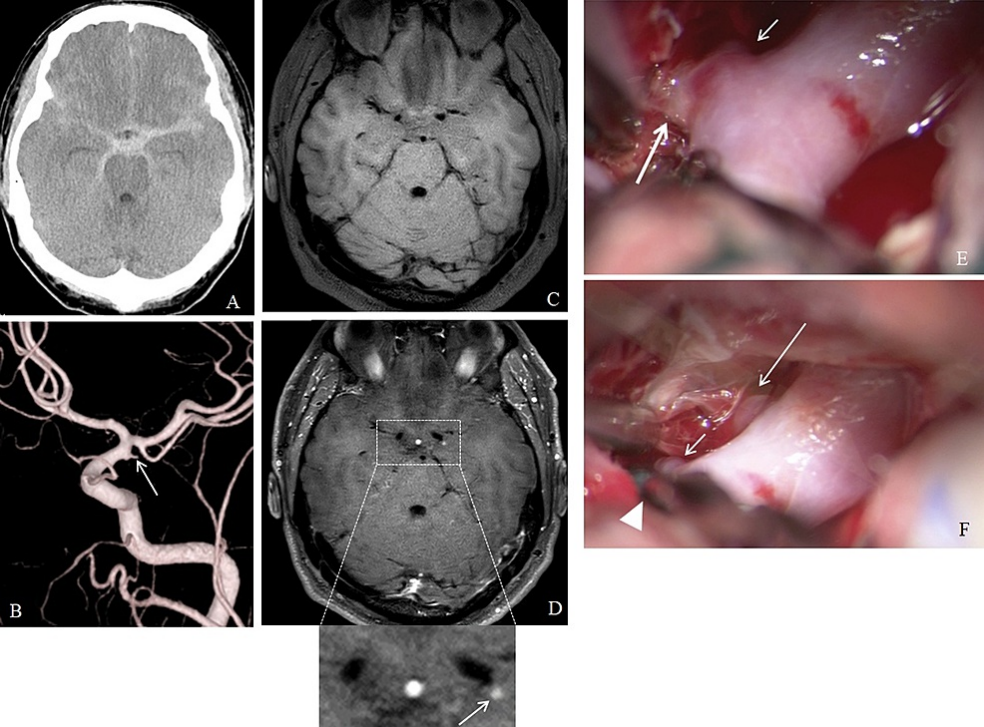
Case 1 (A) Computed tomography shows diffuse subarachnoid hemorrhage. (B) Digital subtraction angiography demonstrates a small aneurysm (arrow). Magnetic resonance vessel wall imagings (C) pre-contrast and (D) post-contrast administration show enhancement of the apex of the aneurysm (arrow). (E, F) Intraoperative photographs show the aneurysm surrounded by subarachnoid hemorrhage (thick arrow: aneurysm, short arrow: anterior choroidal artery, long arrow: posterior communicating artery, arrowhead: mini-clip).
